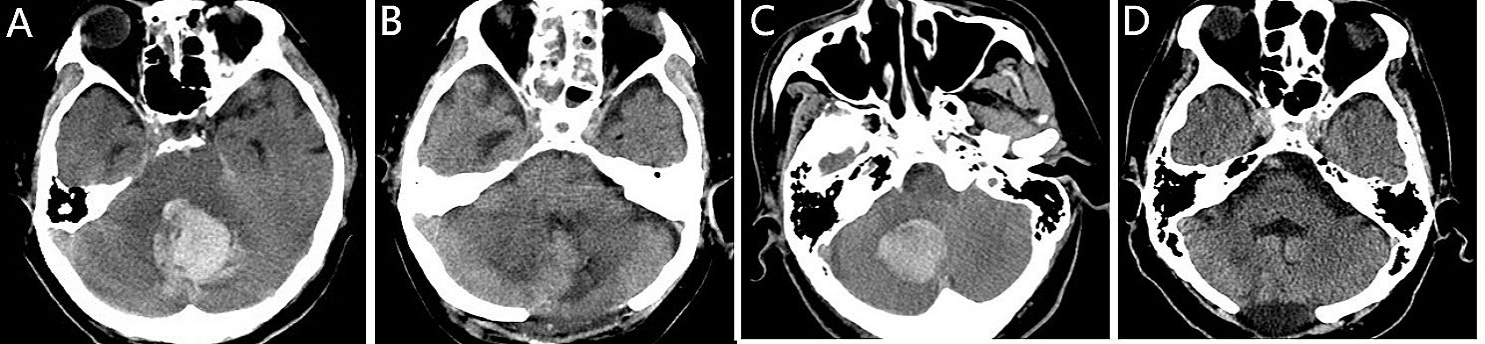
A&B: A 56-year-old male patients with cerebellar hematoma and the CT morphology of the posterior cranial fossa after craniotomy. C&D: A 62-year-old female patients with cerebellar hematoma and the CT morphology of the fourth ventricle and cisterna ambiens after craniotomy
